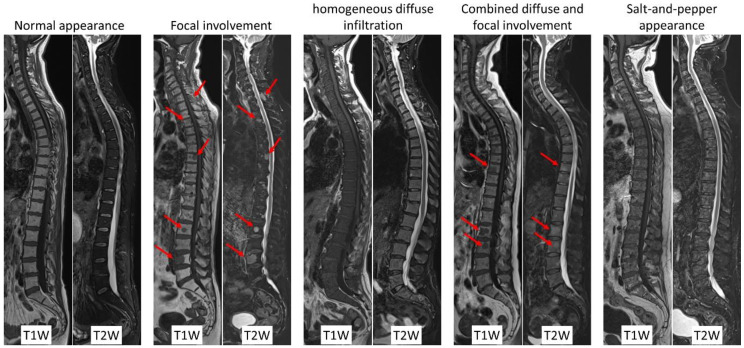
T1-weighted spin-echo and Dixon T2-weighted water-only images of the whole spine in the sagittal plane. Typical MRI findings associated with the five recognized bone marrow infiltration patterns. From left to right—normal appearance of bone marrow, focal lesion or focal involvement (arrows), homogeneous diffuse infiltration, combined diffuse and focal (arrows) involvement, and variegated or salt-and-pepper appearance.
